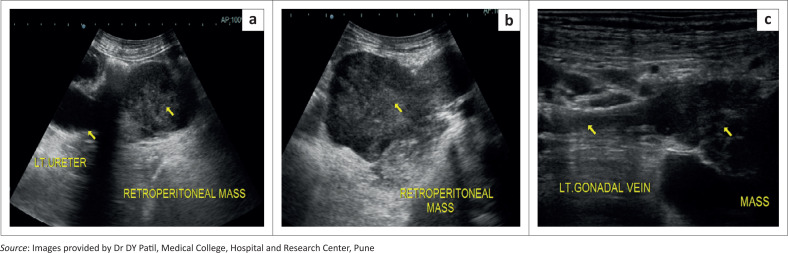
(a–c) Ultrasound abdomen showing retroperitoneal mass of heterogeneous echotexture along the course of the left gonadal vein involving adjoining left ureter with proximal hydroureter.
